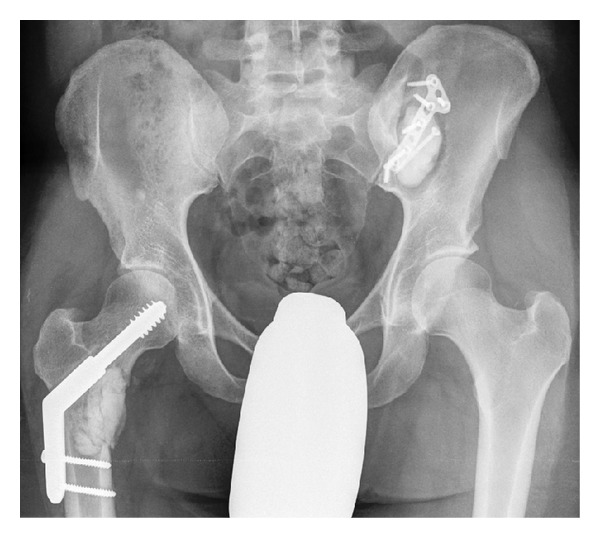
Conventional radiography of the pelvis (AP view): after curettage of the lesions at the right proximal femur and of the left iliac wing, the defects were filled with bone cement; prophylactic stabilizations using a dynamic hip screw at the right side and a plate at the left posterior iliac crest were performed.
